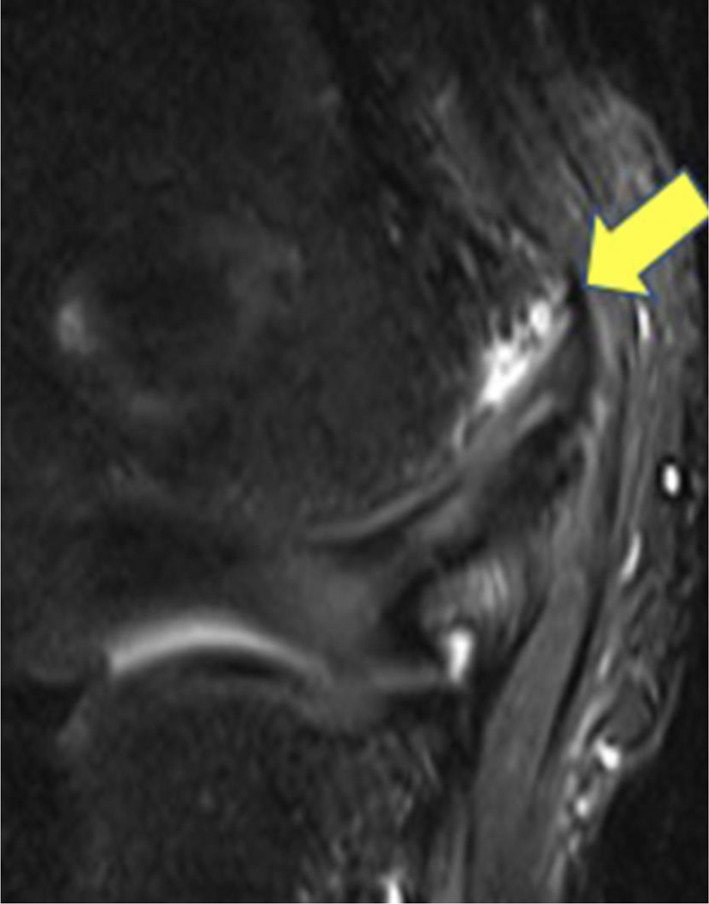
Little League elbow. T2 magnetic resonance image (MRI) demonstrating increased signal intensity at the medial epicondyle apophysis. Reproduced from Kajiwara et al. [49], with permission
